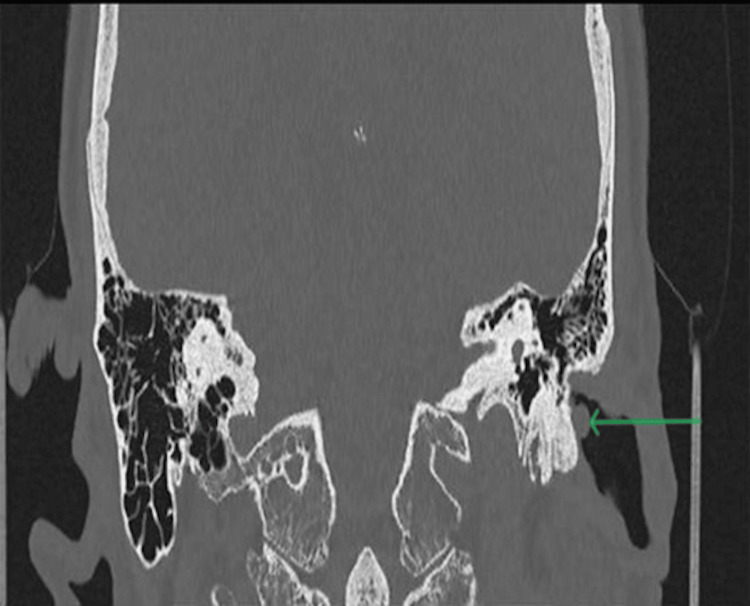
CT scan (coronal view) of the left external auditory canal showing a 6 × 6 mm soft tissue lesion without bony erosion.
